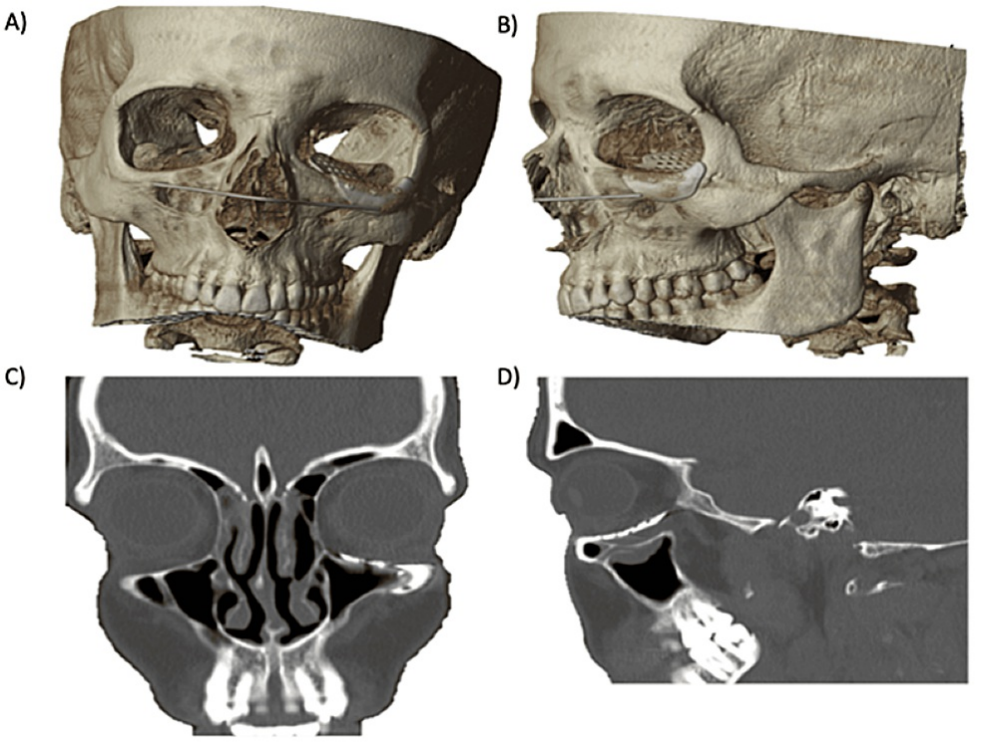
Postoperative CT scan, coronal and sagittal views with 3D reconstruction, showing PSI adaptation. A) 3D reconstruction, frontal view; B) 3D reconstruction, lateral view; C) correction of orbital floor asymmetry in CT coronal slice; and D) PSI adaptation in CT sagittal slice. CT: Computed tomography; 3D: Three-dimensional; PSI: Patient-specific implant
